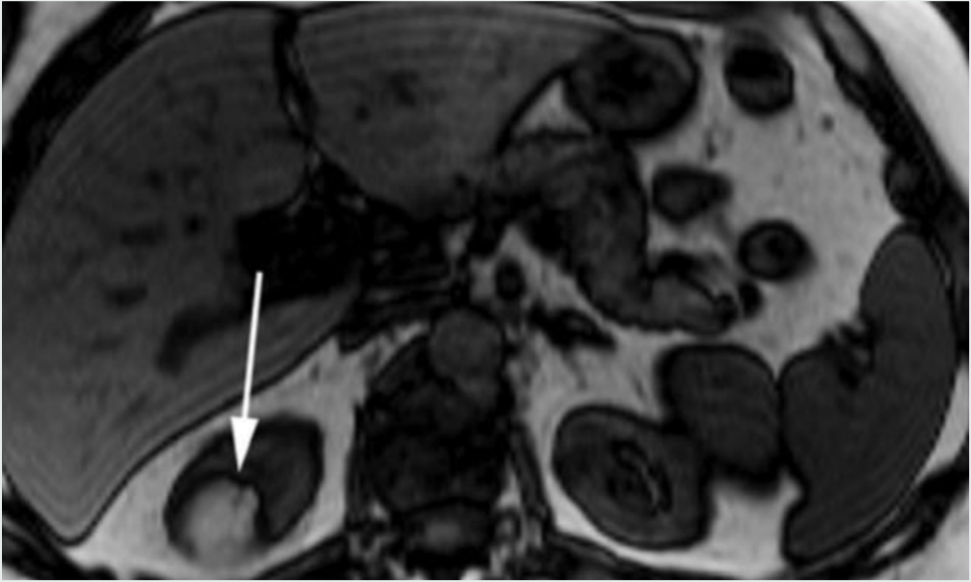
Opposed-phase T1-weighted MR image with a hyperintense renal mass. The India ink artefact (arrow) is present at the interface of the lesion with the kidney [16].
